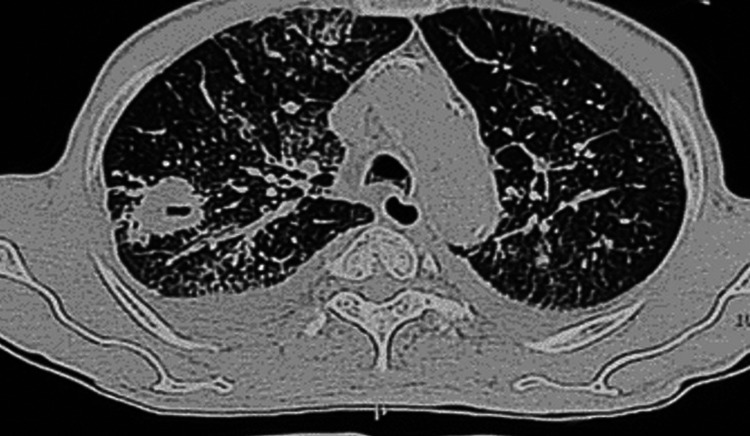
Chest CT showing right upper lobe cavitation along with bilateral lung nodules. Right moderate and left mild pleural effusion is also seen.
